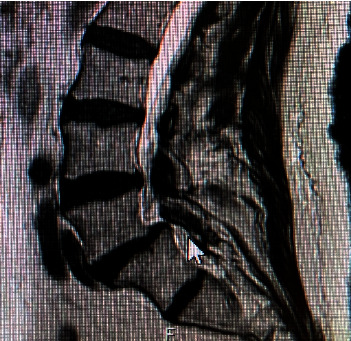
MRI lumbar spine with contrast showing grade 1 spondylolisthesis at L5–S1 with S1-S2 disk space causing severe spinal stenosis. There is a large object extending inferiorly from L5–S1 disk space.
